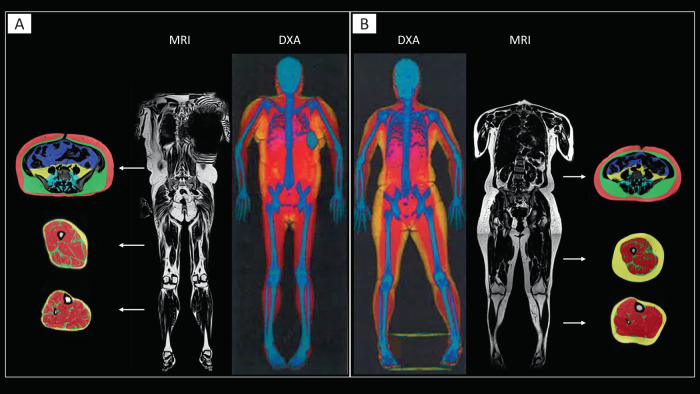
MRI and DXA images of a female patient with partial lipodystrophy and a control with identical BMI. Representative MRI and DXA images from a female patient A with FPLD and B a control subject who have identical BMI (27 kg/m^2^). The FPLD patient A showed markedly different body fat distribution with higher abdominal deep subcutaneous adipose tissue (green), superficial subcutaneous adipose tissue (red), intraperitoneal adipose tissue (blue), retroperitoneal adipose tissue (yellow), and paraspinal adipose tissue (cyan), and selective loss of SAT in the thigh and calf compared to the control subject B. The artifact in the MRI image A is due to an implantable cardioverter defibrillator.
